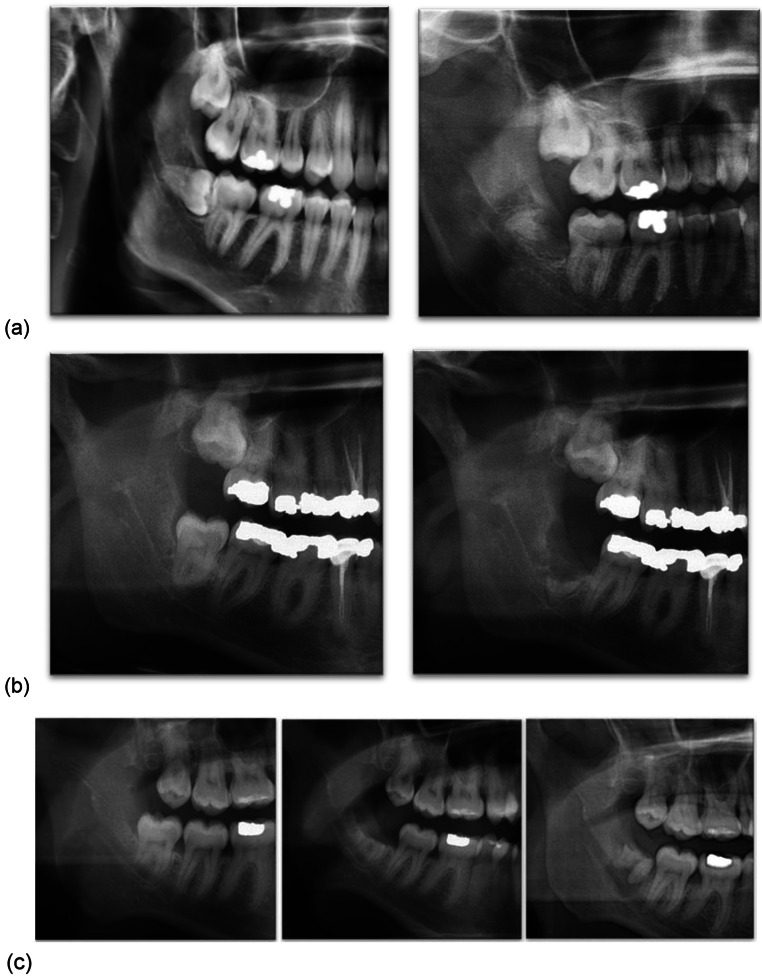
Orthopantomograms of cases requiring re-operation. (a) Case 1, (b) Case 2 (c) Case 3 demonstrating root migration
